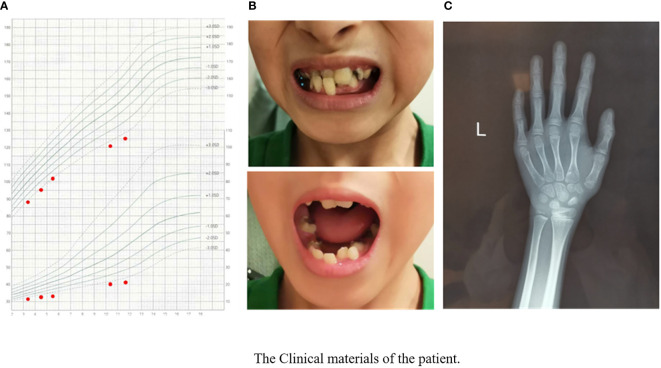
The clinical materials of the patient’s growth chart. (A) The manifestation of the patient’s teeth. (B) X-ray of the patient’s left hand and wrist. (C) Bone age was approximately 7 years when the patients’ chronological age was 11 years 8 months.
